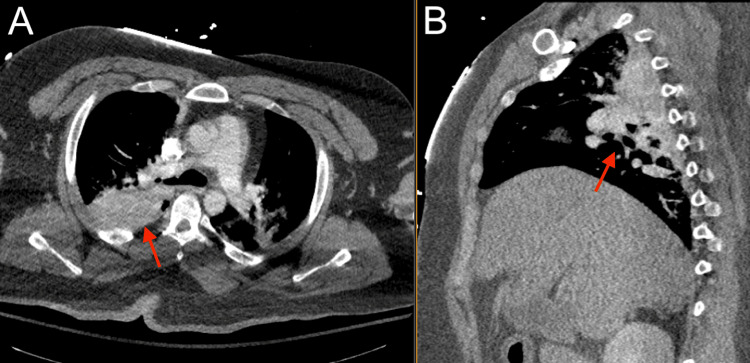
Chest CTA showing possible pulmonary hemorrhage, contusion, and atelectasis (red). (A) Axial view; (B) Sagittal view CTA: computed tomography angiogram
